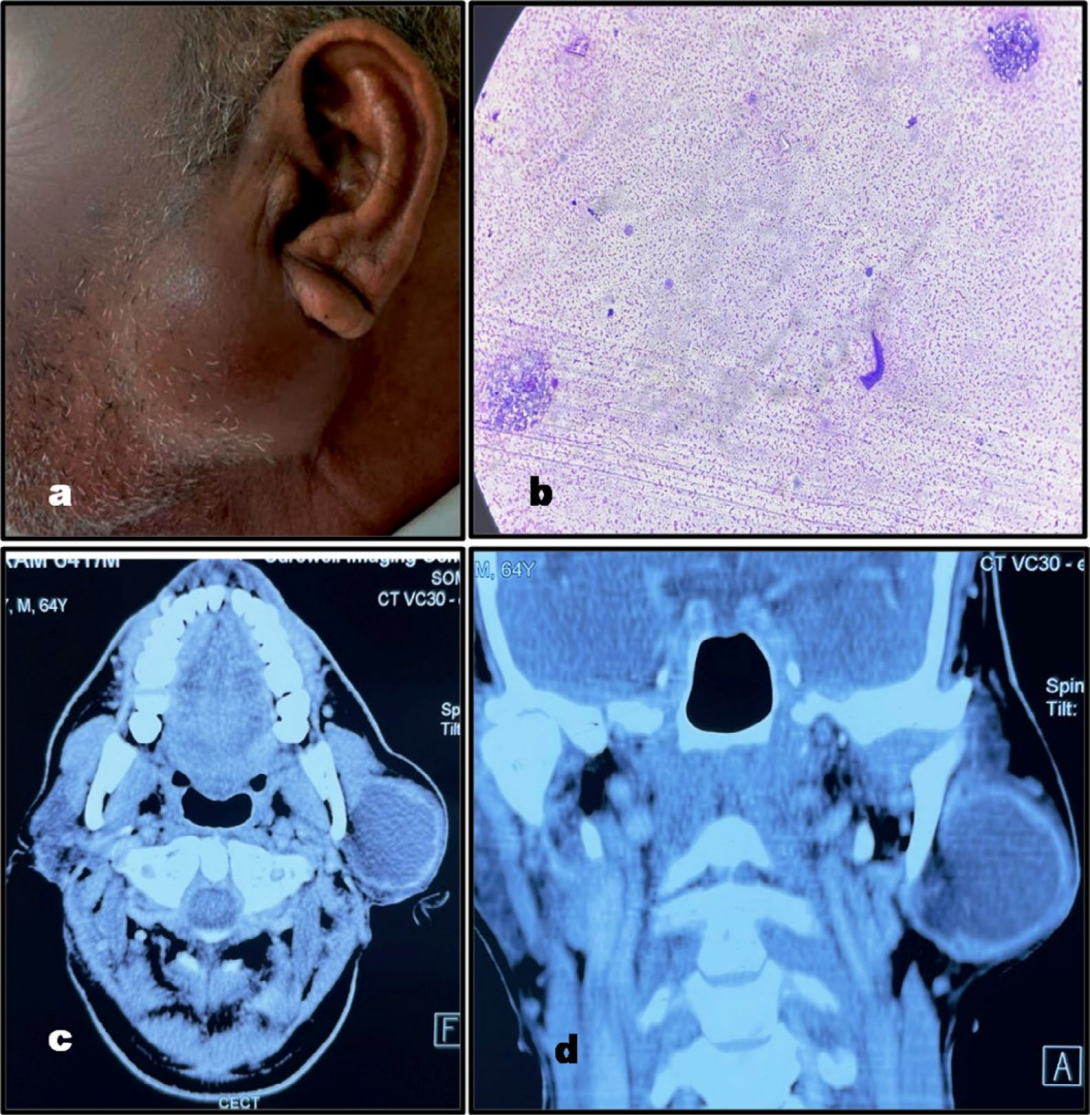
Pre‐operative presentation of the pathological swelling: (a) Clinical view. (b) cytological smear‐cystic fluid with scanty macrophages (100×). (c) CT scan axial view—showing left parotid swelling. (d) CT scan coronal view showing left parotid swelling.
